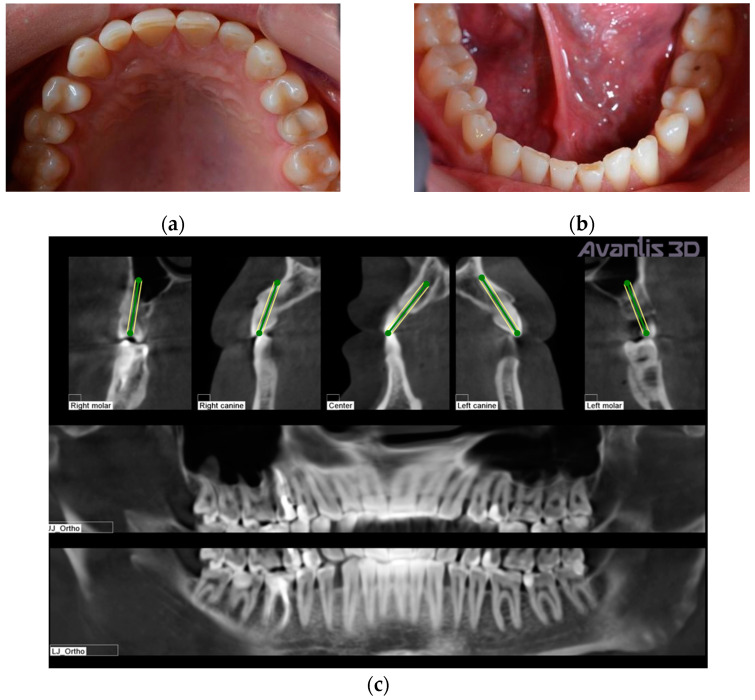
Intraoral occlusal views: (a) upper dental arch; (b) lower dental arch; (c) X-ray before treatment.
